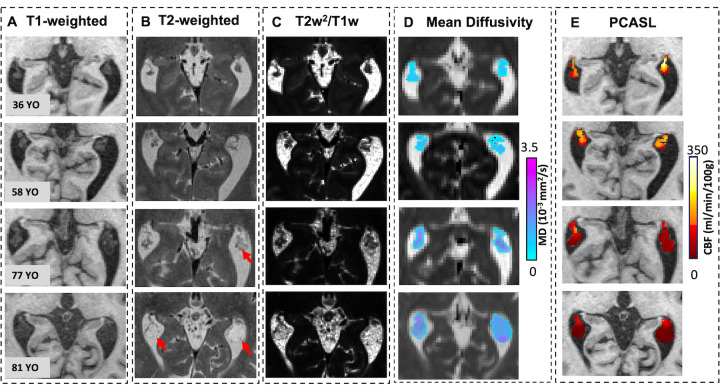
Age-related alterations of the choroid plexus in different MRI modalities. (A) With aging, the ChP volume is getting large on T1-weighted MRI without contrast agent. (B) In T2-weighted MRI, the ChP is hypointense and becomes less dense with aging. (C) The T2w2/T1w ratio mapping enhances the contrast between vessel and non-vessel compartment of ChP; (D) The outline of ChP can be better delineated in the mean diffusivity (MD) map, where MD values increase with age within the ChP. (E) The CBF of the ChP is lower in the aged population than young population. Adapted from Ref [114].
